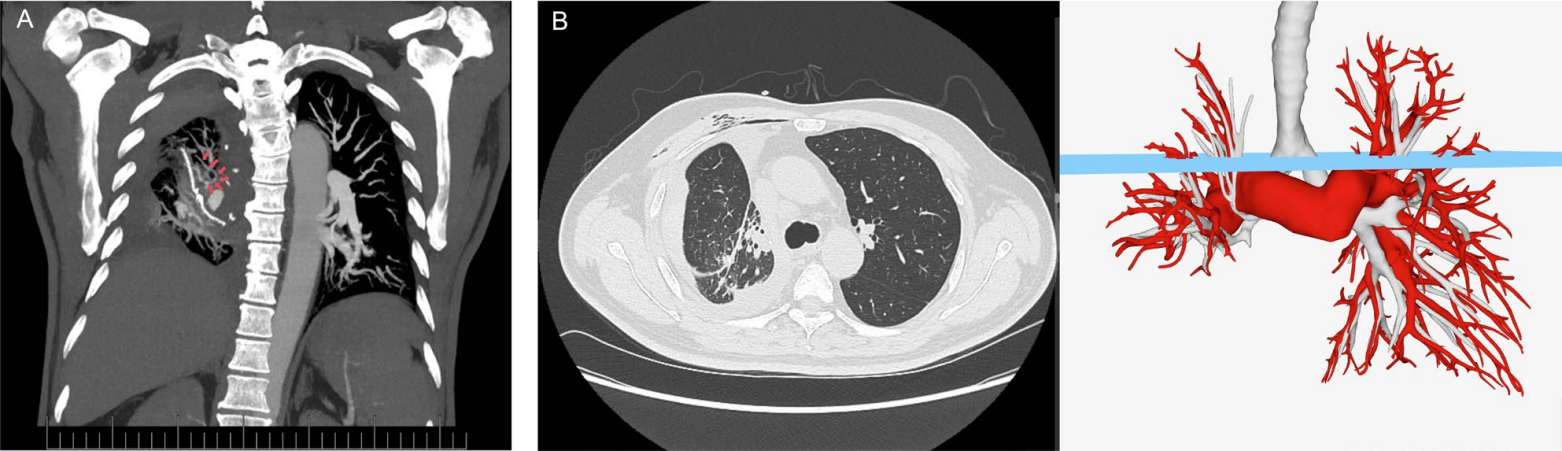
CT imaging and 3D reconstruction of pulmonary vasculature and airways after 3 days of conservative treatment. (A) Mild residual vascular swirling in the distal right lung vasculature (arrow). (B) Despite these findings, axial CT at the previously obstructed level and corresponding 3D reconstruction model demonstrate good distal opacification with significant resolution of atelectasis
